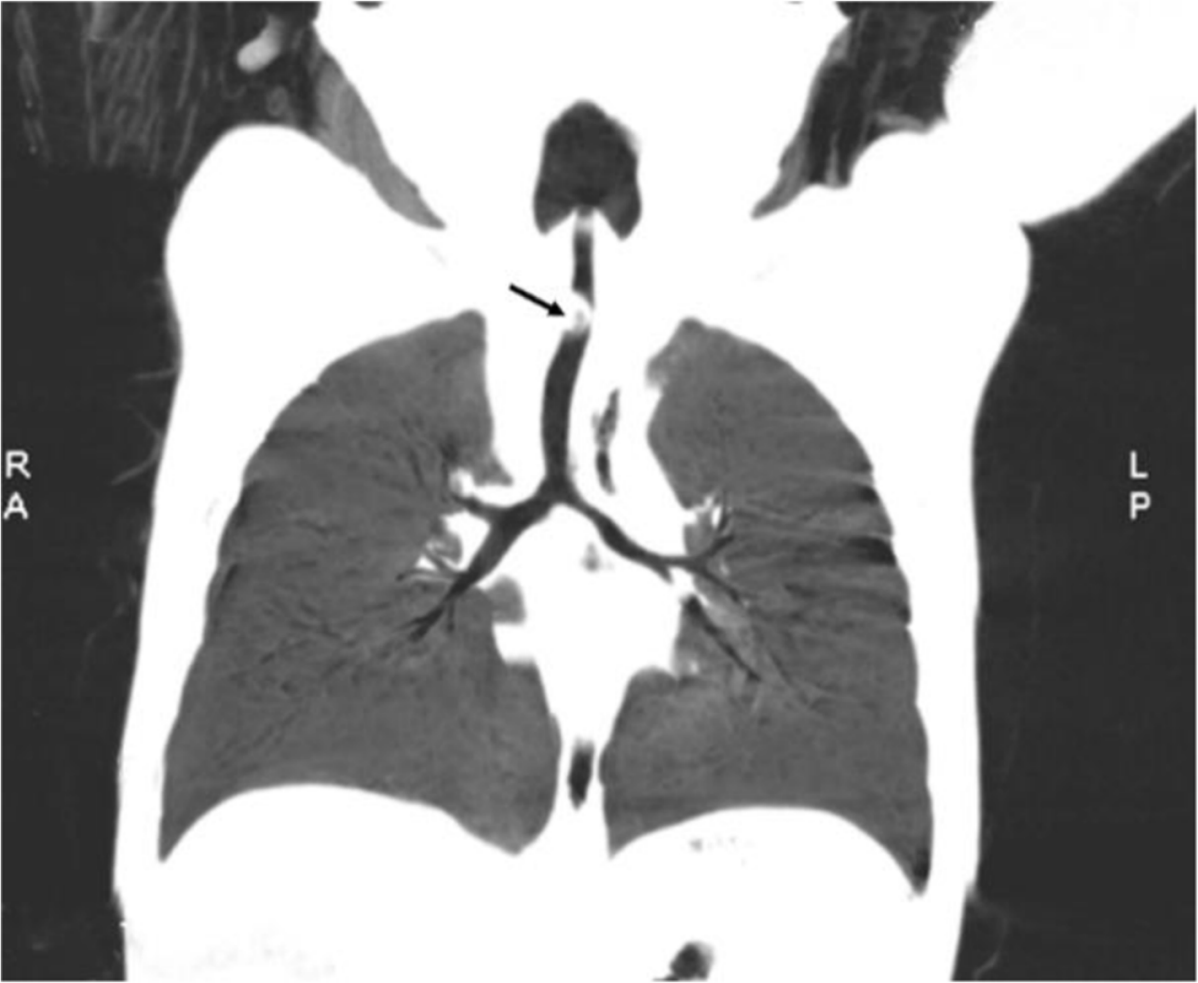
Multislice spiral CT showed an endotracheal foreign body (peanut)
